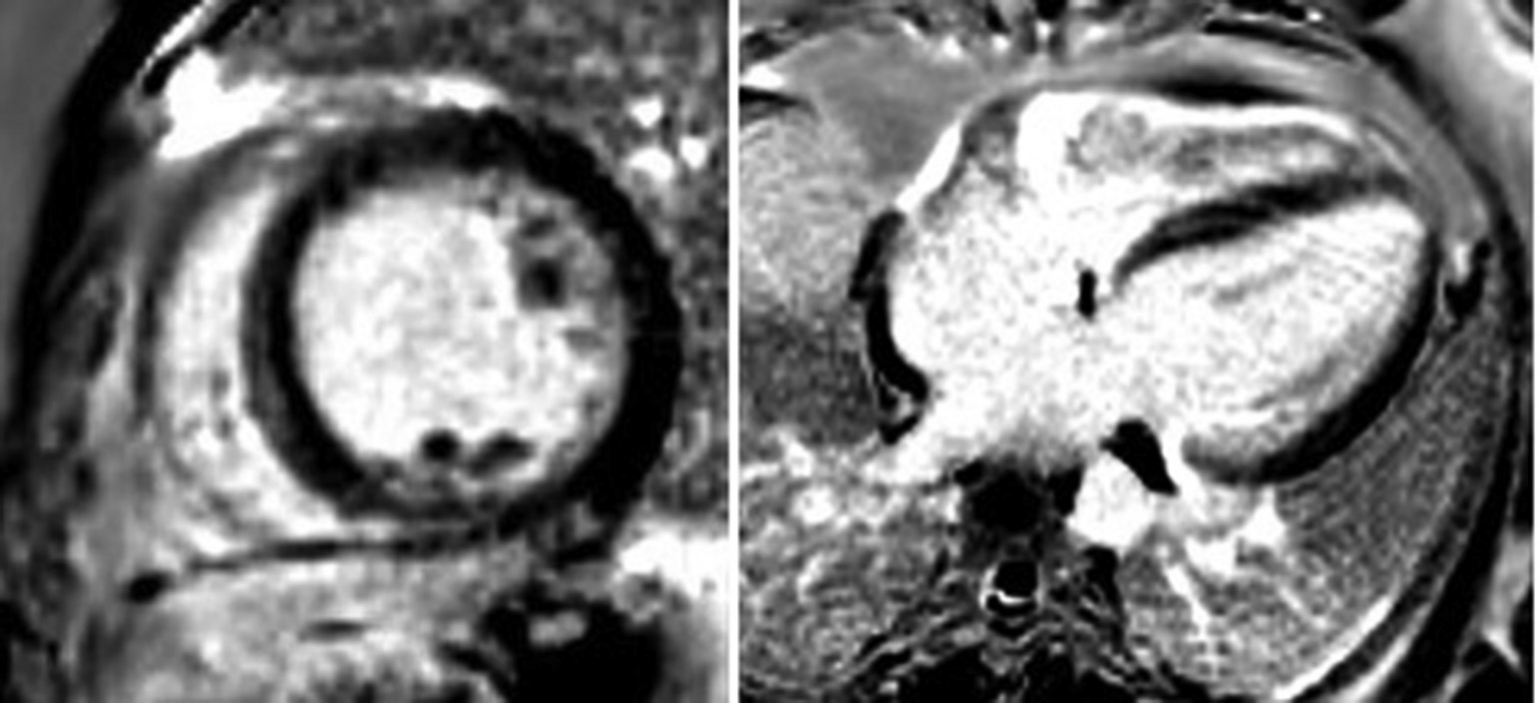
Cardiac magnetic resonance imaging with short axis at the level of the papillary muscle (*left*) and long axis 4-chamber (*right*) demonstrating no evidence of late gadolinium enhancement.
